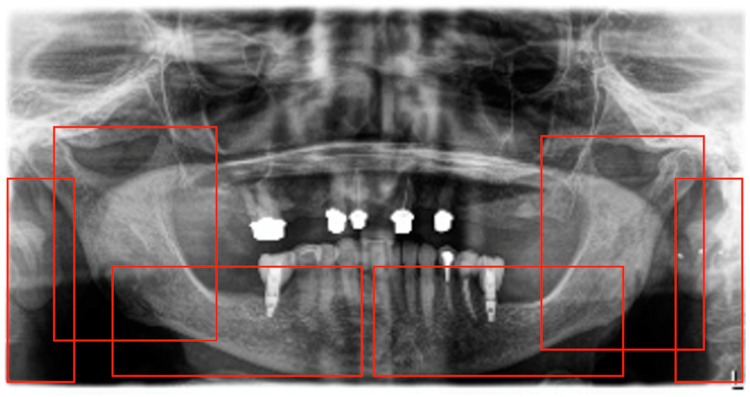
In each panoramic X-ray (PR), six regions of interest were identified: the left and right projections of the vertebral spine, the left and right posterior regions of the mandible (including the angulus, ramus, condyle, and coronoid process), and the left and right anterior regions of the mandible, extending from the symphysis up to the angulus.
